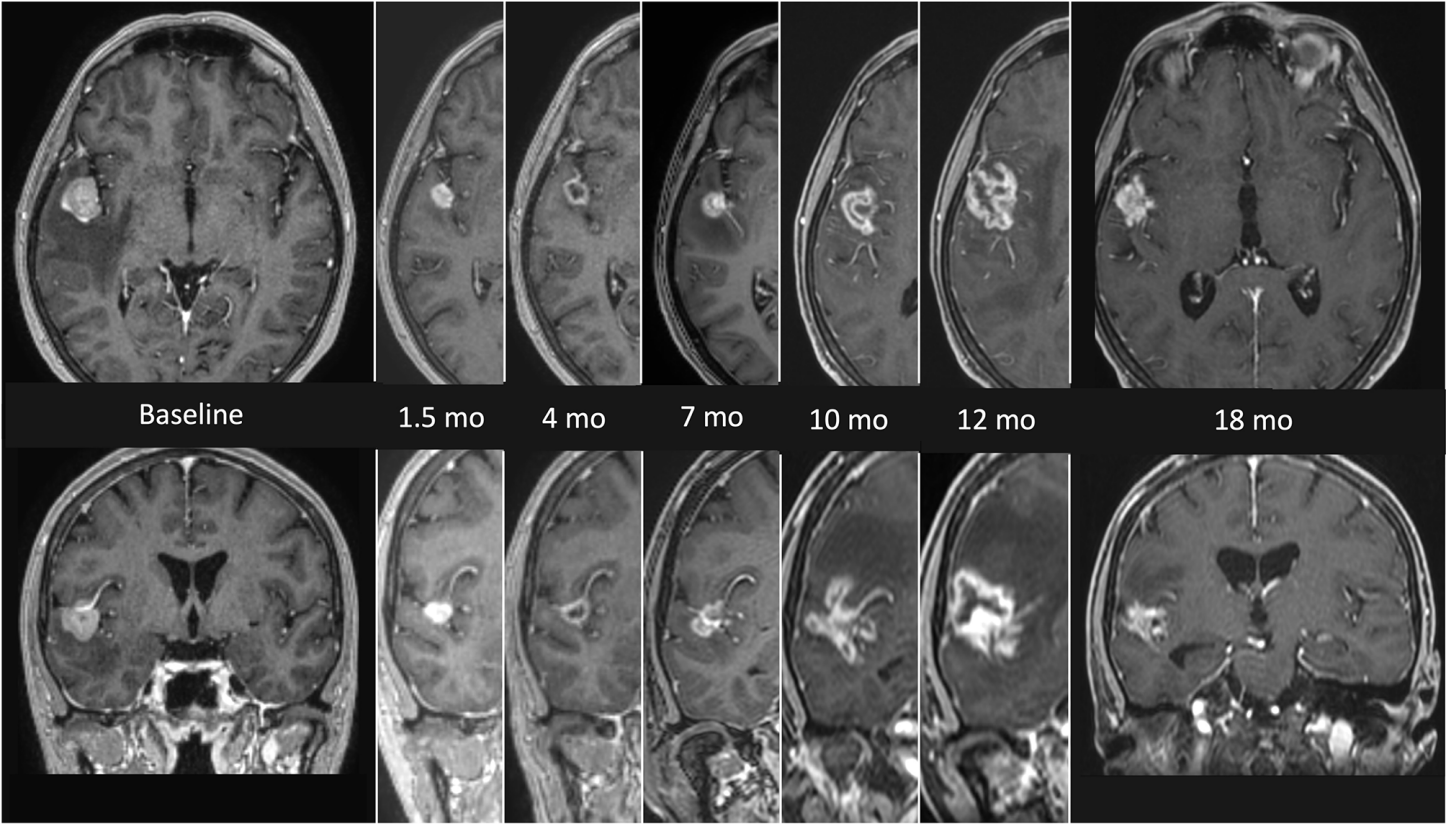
MRI findings presenting the evolution of radionecrosis (RN) within a timeframe of 18 months from the end of radiosurgery (SRS) in a single right temporal BM from NSCLC. The first images on the left (axial and coronal) represent the lesion before focal treatment. Serial follow up images show an initial shrinkage of the treated lesion followed by a significant and continuous increase of the necrotic component. The latest images on the right (axial and coronal) refers to the situation 18 months after SRS, with a clear reduction of the necrotic component, which allows a differential diagnosis with a relapse.
